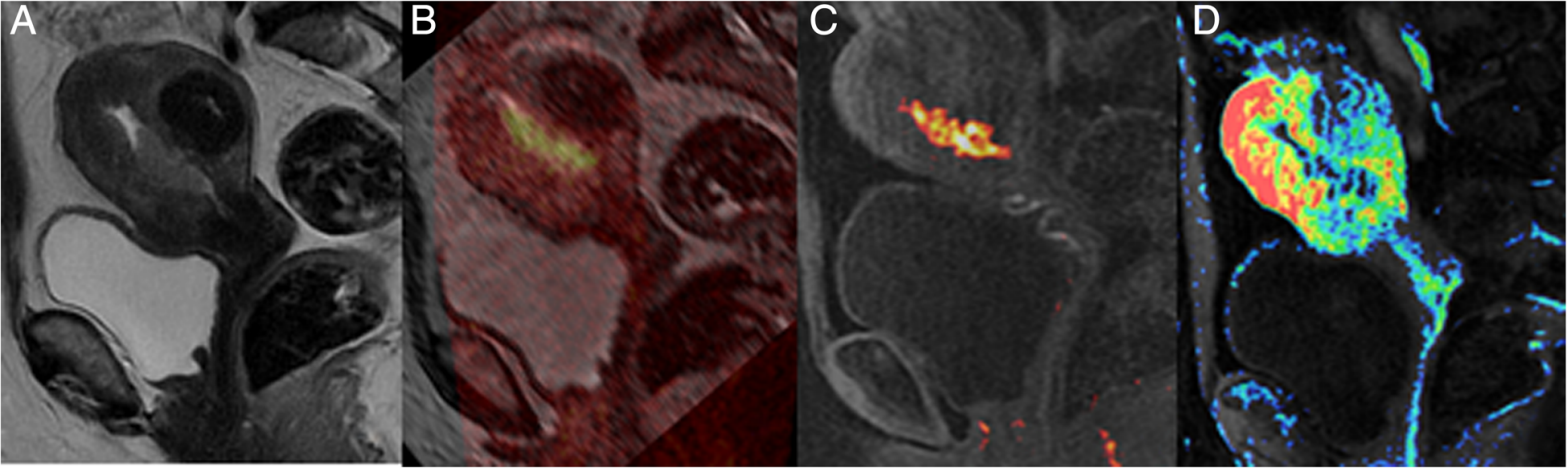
Images of a 56-year-old woman with biopsy proved prostate cancer: multiparametric study: T2WI (a). FOCUS DWI images of b = 1000 s/mm2 (b and c). Perfusion map T2WI on sagittal plane (d) shows endometrial lesion confined to the endometrial cavity. FOCUS DWI images show tumor lesion in higher spatial resolution with a definite clear border, less artifacts, and image blurring
